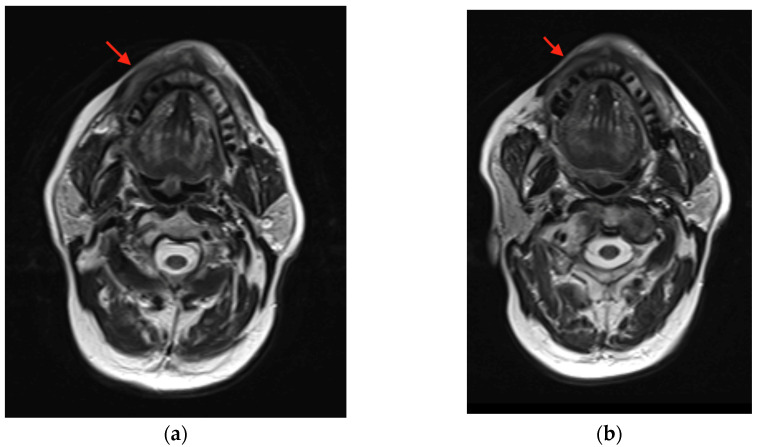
MRI shows (a) buccal thickening (red arrow) in site of carcinoma pretreatment and (b) interval decreased thickening (red arrow) of right buccal tissues post treatment.
